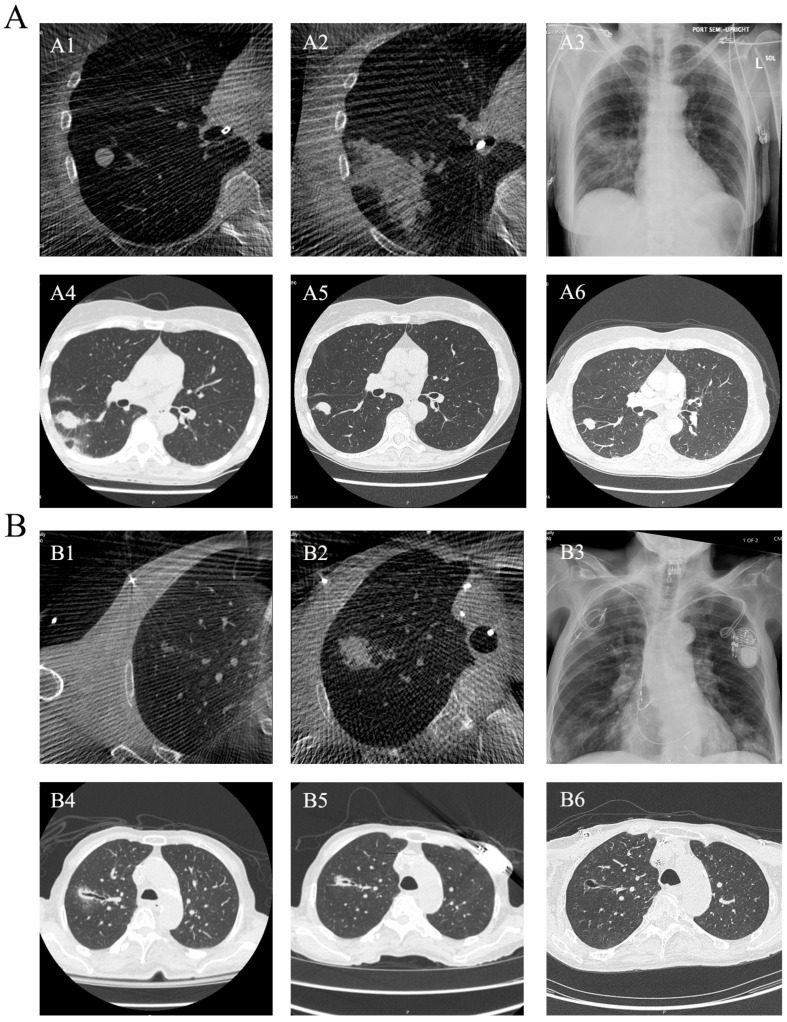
Representative temporal imaging evolution following ssRAB-MWA during the first two months. (A) Serial CT/CBCT images from Case 2 demonstrating lesion appearance before MWA (A1), immediately after MWA (A2), 1 h post-MWA (A3), 1 day post-MWA (A4), 22 days post-MWA (A5), and 56 days post-MWA (A6). (B) Serial CT/CBCT images from Case 8 demonstrating lesion appearance before MWA (B1), immediately after MWA (B2), 1 h post-MWA (B3), 2 days post-MWA (B4), 12 days post-MWA (B5), and 55 days post-MWA (B6).
